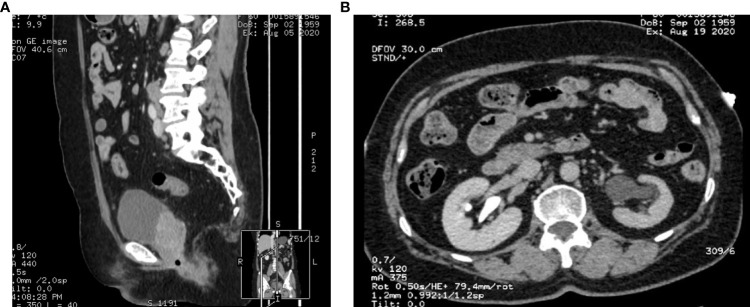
(A, B) Abdominal computed tomography (August 5, 2020).
